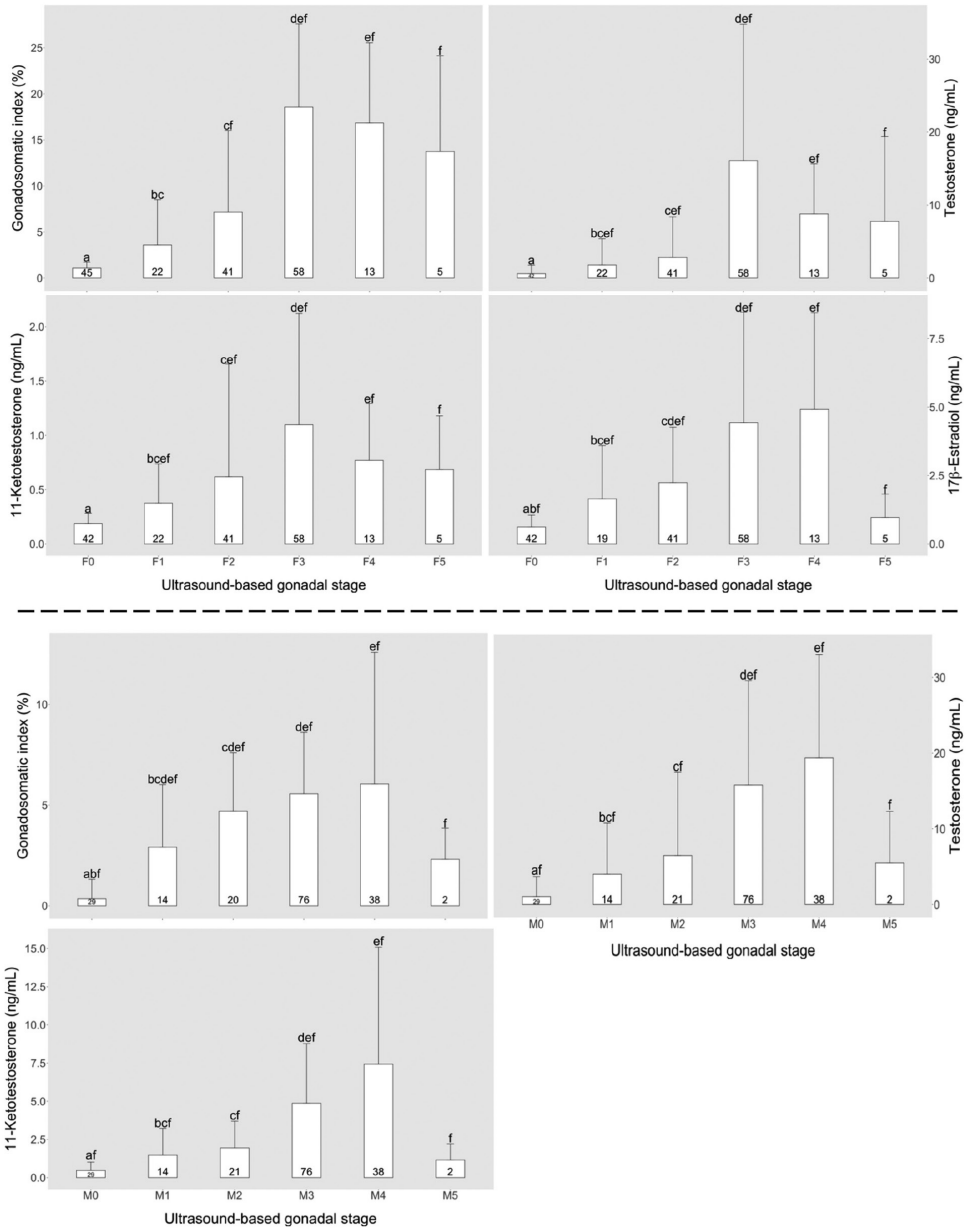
Gonadosomatic index and plasma concentrations of sex steroids at different ultrasound gonadal stages in lumpfish females (top) and males (bottom). Each ultrasound stage is assigned a unique letter (F0 & M0 = a, F1 & M1 = b, F2 & M2 = c, F3 & M3 = d, F4 & M4 = e, F5 & M5 = f), thus where an ultrasound stage carries the same letter as the next ultrasound category, it implies that difference between the two is not statistically significant. The data are presented as mean ± SD. Values inside bars represent number of fish in each ultrasound stage.
